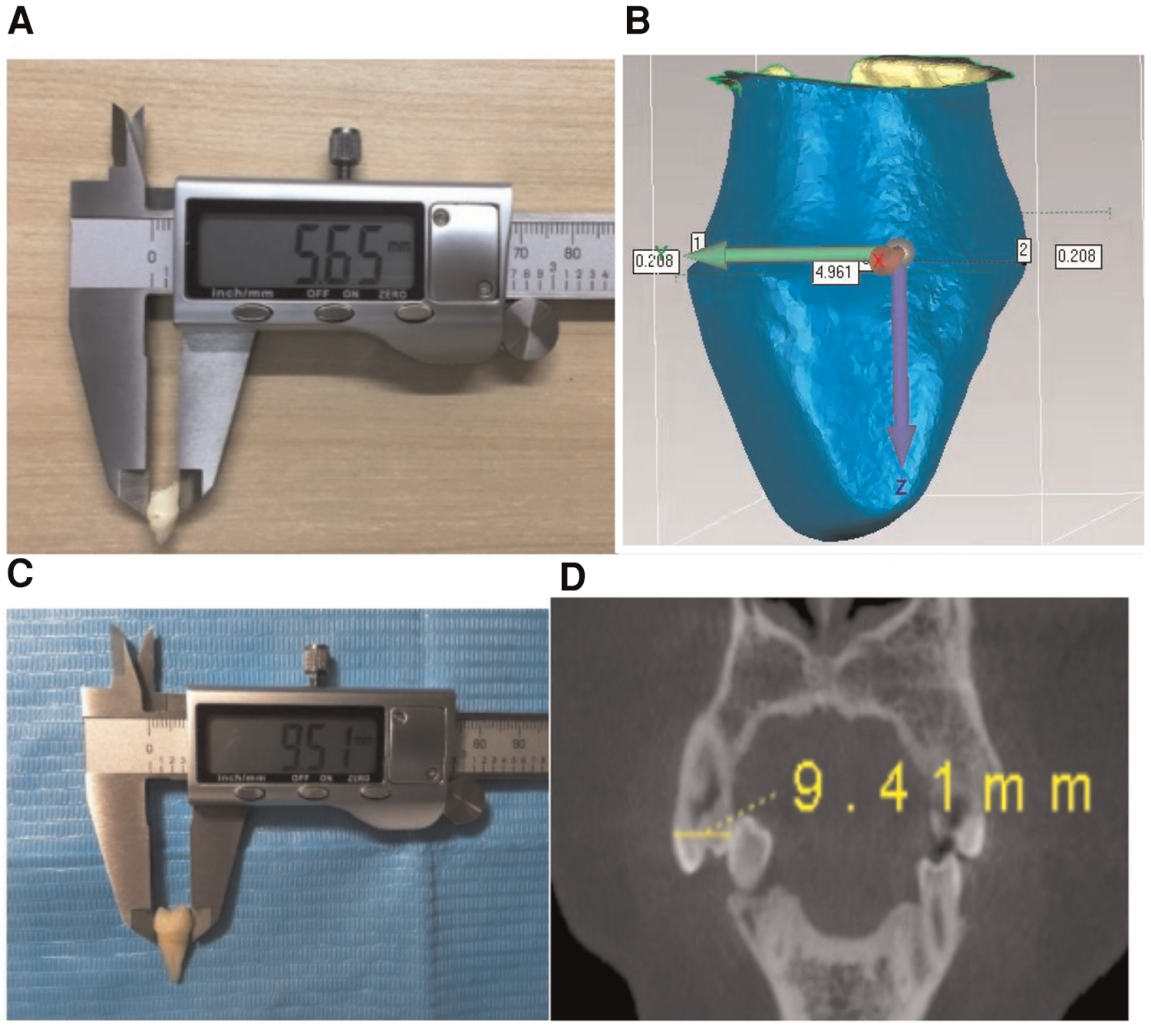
(A) Manual measurement of extracted primary teeth with a Vernier calliper. (B) Digital measurement of extracted primary teeth with an intraoral scanner. (C) Manual measurement of extracted maxillary first premolar with a Vernier calliper. (D) CBCT image digital measurement of maxillary first premolars.
